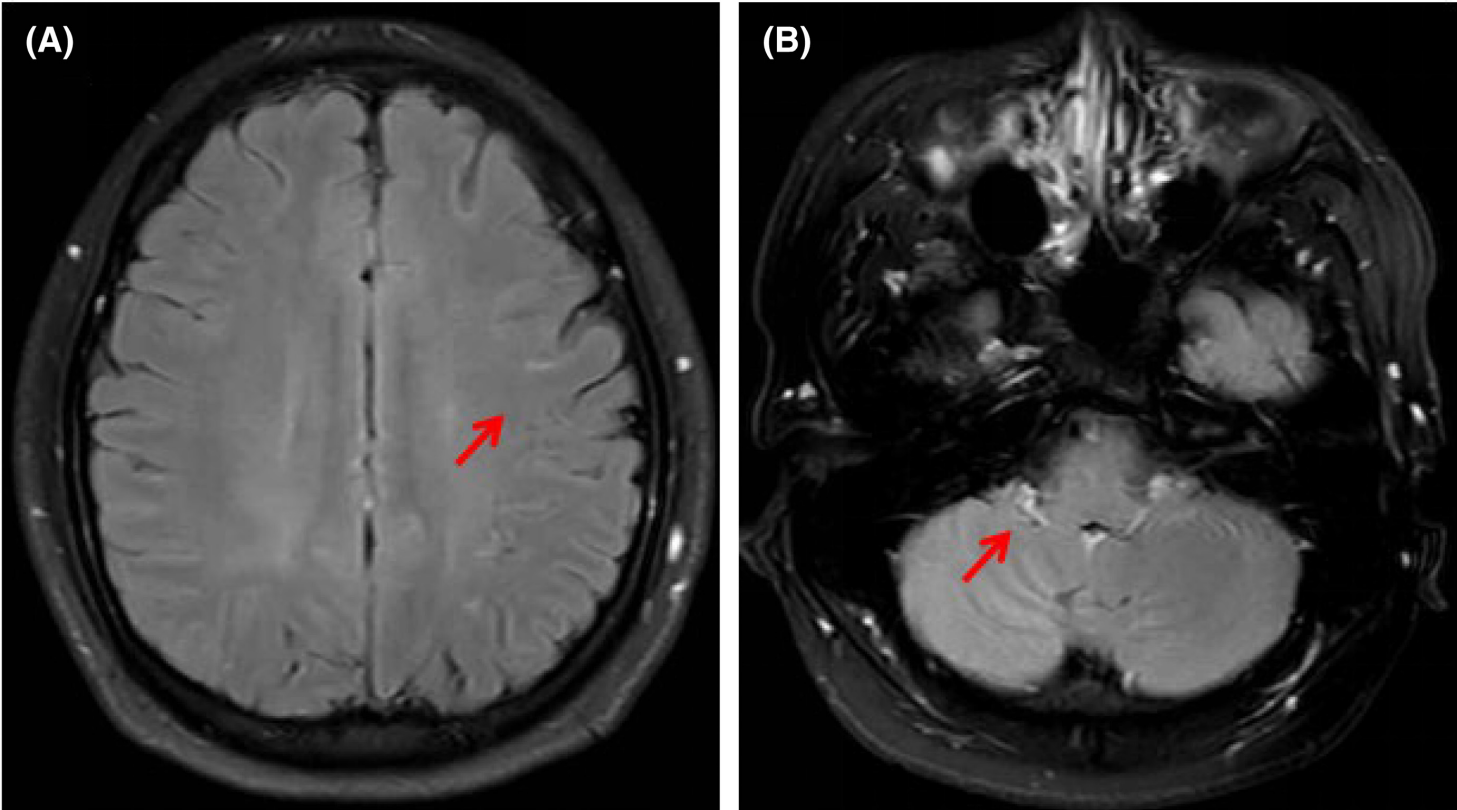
(A) Contrast‐enhanced T2‐weighted magnetic resonance imaging (T2WI) shows that leptomeningeal appears as enhancing curvilinear segments following the gyral convolutions of the bilateral cerebral hemispheres (arrow). (B) Contrast‐enhanced T2WI FLAIR showing the significant enhancement of the bilateral cerebellar leptomeningeal (arrow).
